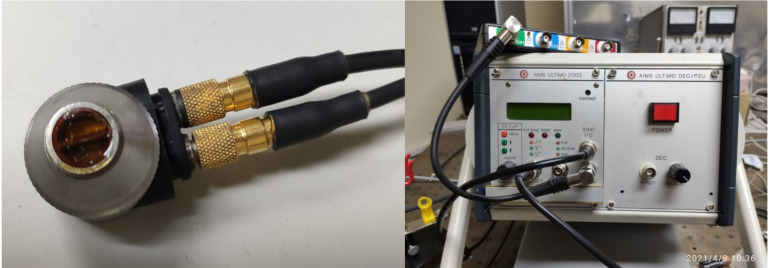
Ultrasonic transducer (left) and equipment (right) used for the experiments. The properties of the ultrasonic pulses (intensity and frequency) that arrive at the interface between the transducer surface and the drop samples depend on the settings of the ultrasonic pulser, the oscilloscope and the transducer characteristics.
